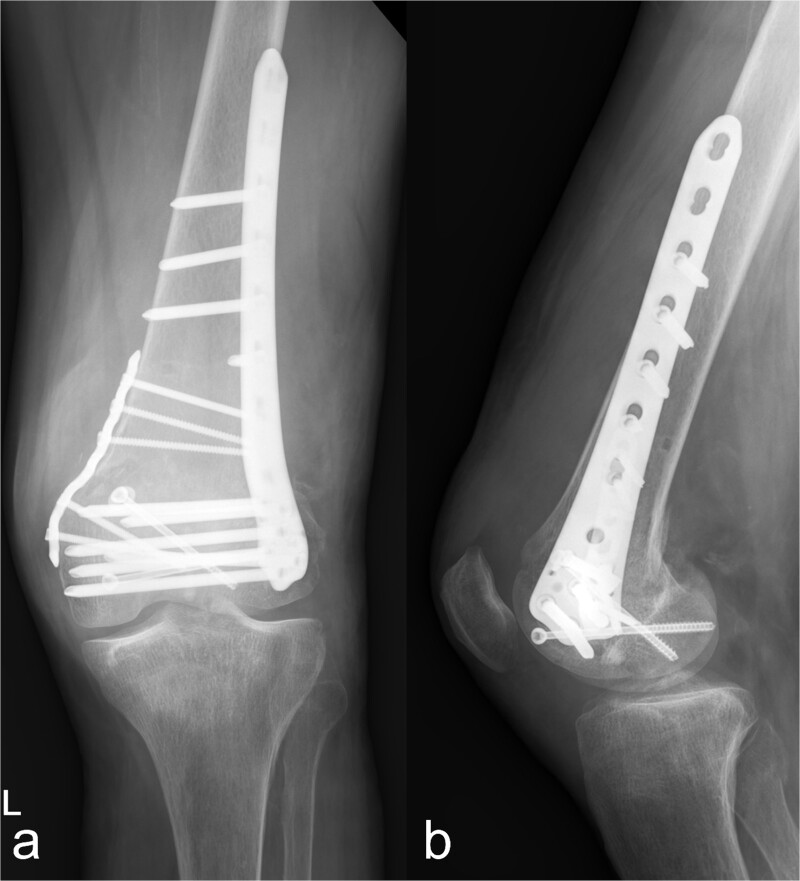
Treatment patterns of supra-intracondylar multifragment fracture (33C3.2). (a) The anterior-posterior and (b) lateral radiographs show a correct position of the osteosynthesis material with an nearly anatomical fracture position.
